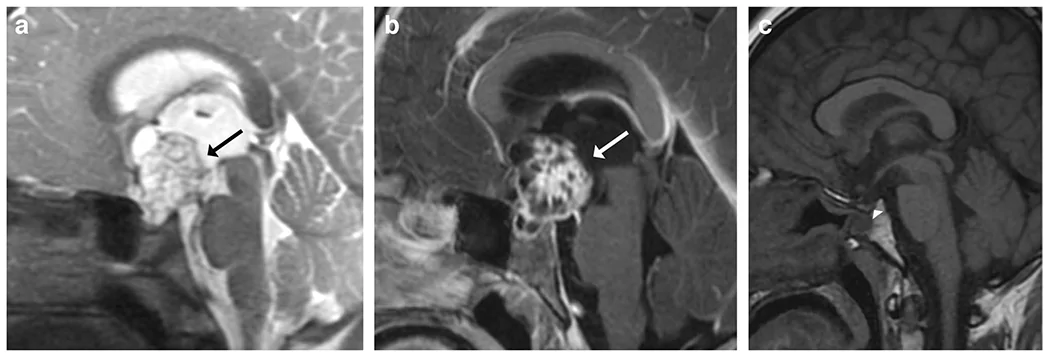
Fifteen-year-old male with treated adamantinomatous craniopharyngioma discovered at age 6 years for headache evaluation and multiple resulting endocrine abnormalities, including adrenal insufficiency, growth hormone deficiency, central hypothyroidism, gonadotropin deficiency, and diabetes insipidus. a Initial sagittal volumetric T2-weighted sequence and (b) sagittal post-contrast T1-weighted image through the midline show a mixed cystic and solid lobulated mass in the sella and suprasellar space (*arrows*). c Follow-up sagittal T1-weighted sequence shows interval resection of the tumor via a transsphenoidal approach. No discernible pituitary infundibulum. Thin pituitary tissue is identified in the sella (*arrowhead*)
